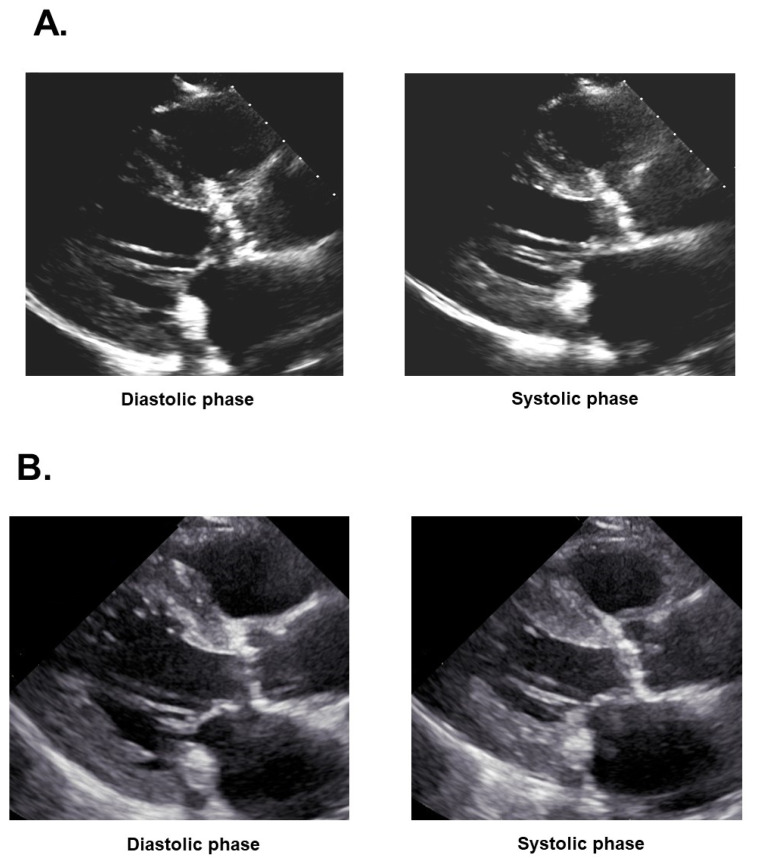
Transthoracic echocardiography (long-axis view) at diastolic/systolic phase on admission expressing left ventricular ejection fraction of 25% (A) and those following 3-month medical therapy showing left ventricular ejection fraction of 36% (B).
